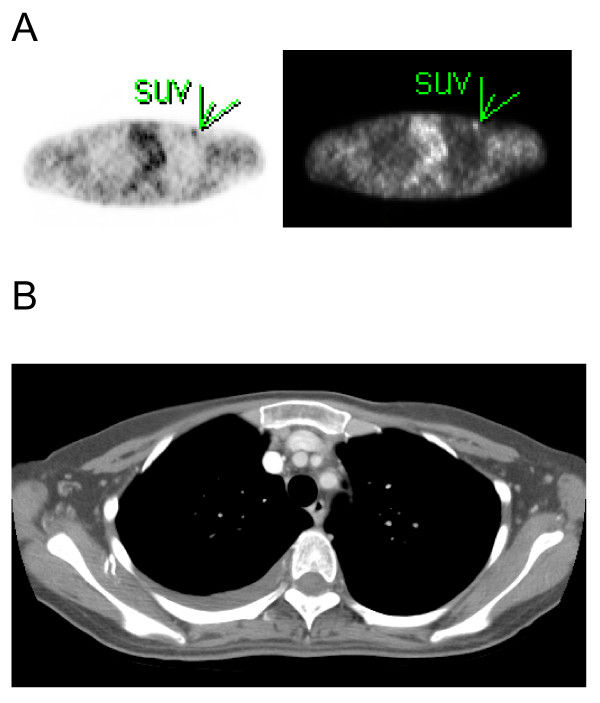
(a) Metastatic lesion in chest wall was markedly positive for uptake with tracer (arrow). (b) The corresponding computed tomographic image reveals that no lesion can be identified.
